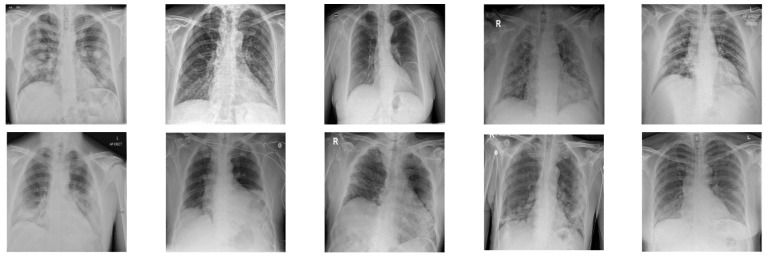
X-ray images used in the proposed method.
